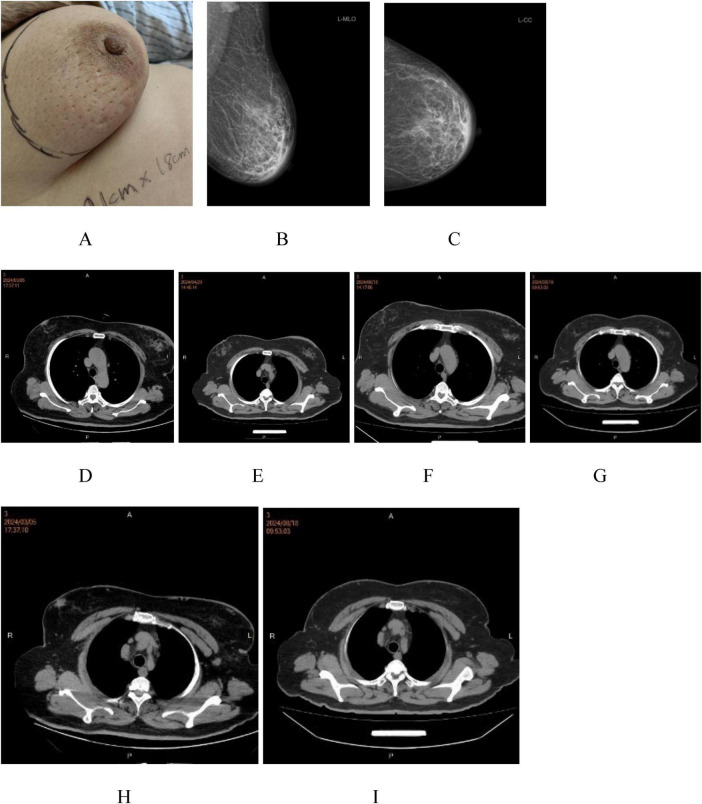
Appearance of the breasts, mammography at initial diagnosis, and computed tomography (CT) images. (A) Photograph of the breasts at initial diagnosis. (B) Mammography at initial diagnosis (mediolateral oblique view, MLO). (C) Mammography at initial diagnosis (craniocaudal view, CC). Mammography revealed central breast skin edema and thickening, with segmental microcalcifications spanning approximately 5.0 cm × 3.5 cm. (D) CT mediastinal window at initial diagnosis (5 March 2024) showed a hyperdense shadow in the left breast measuring approximately 60 mm × 25 mm, with skin edema and thickening. (E) After two cycles of the THP regimen (29 April 2024), CT showed the lesion measuring approximately 53.4 mm × 24 mm, with persistent skin edema and thickening. (F) After two cycles of the THPy regimen (18 June 2024), CT revealed a hyperdense shadow measuring approximately 33 mm × 19 mm, with resolution of skin edema. (G) After four cycles of the THPy regimen (18 August 2024), CT showed a slightly hyperdense shadow measuring approximately 23 mm × 12 mm, with no evidence of skin edema. (H) CT mediastinal window at initial diagnosis showed enlarged axillary lymph nodes. (I) CT mediastinal window on 18 August 2024 showed no abnormal lymph node shadow.
